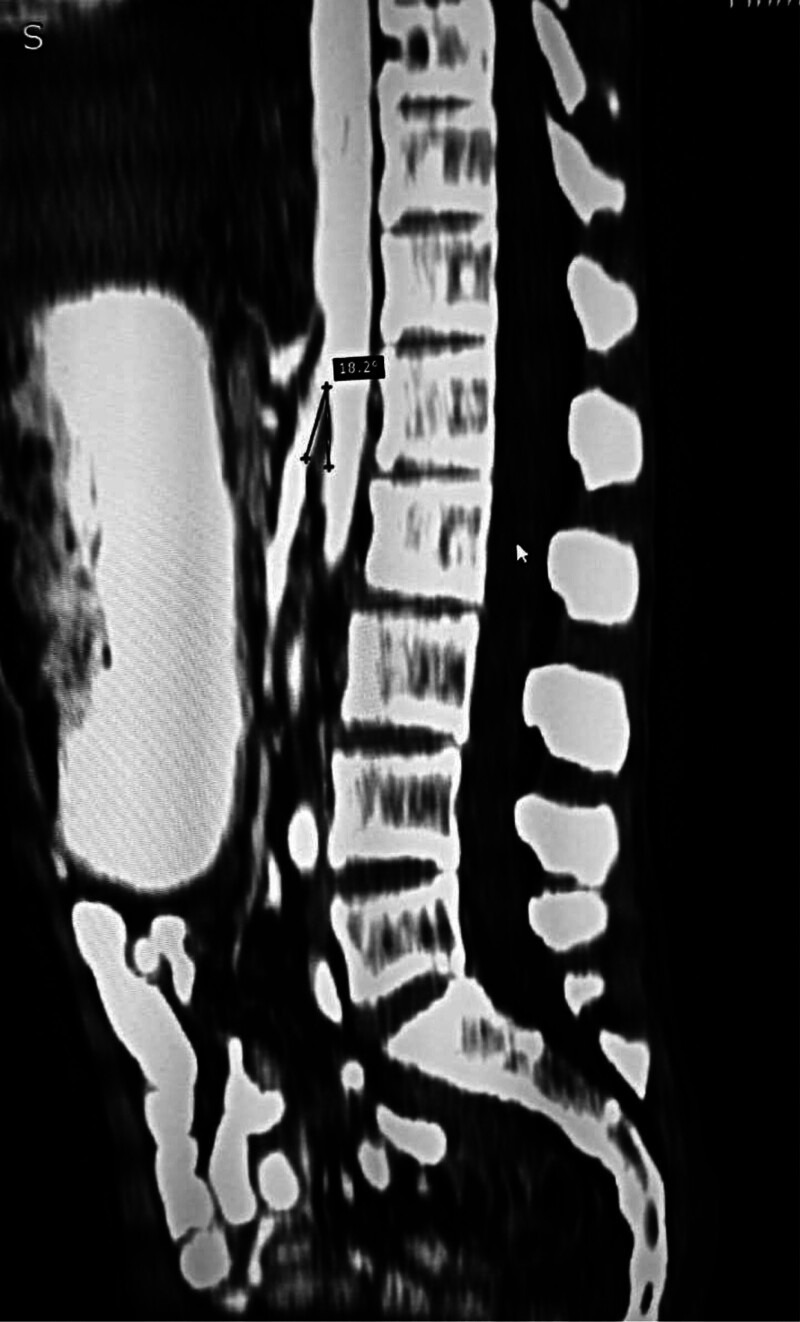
Contrast-enhanced CT scan of the abdomen showing an angle of 18.2° between the superior mesenteric artery (SMA) and the aorta. CT = computed tomography.
